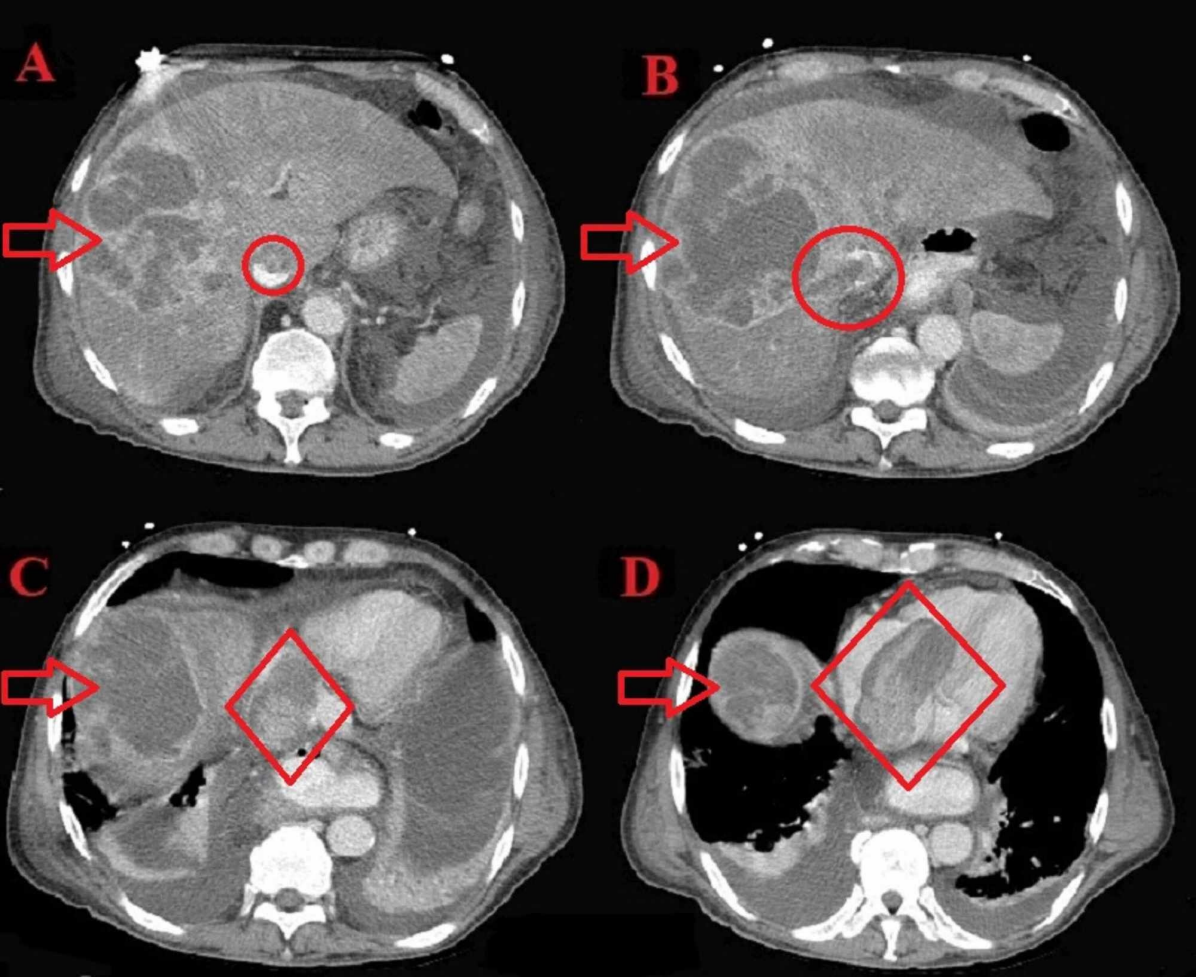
CT scans showing the mass in the liver, right hepatic vein, IVC, RA, and RV. (A) The arrow shows the mass in the liver. The circle shows a filling defect in the IVC suggestive of a mass present in the IVC.(B) The arrow shows the mass in the liver. The circle shows a filling defect extending to the IVC through the right hepatic vein. (C) The arrow shows the mass in the liver. The diamond shows a filling defect in the heart (RA). (D) The arrow shows the mass in the liver. The diamond shows a filling defect in the heart (RV). IVC: inferior vena cava, RA: right atrium, RV: right ventricle
